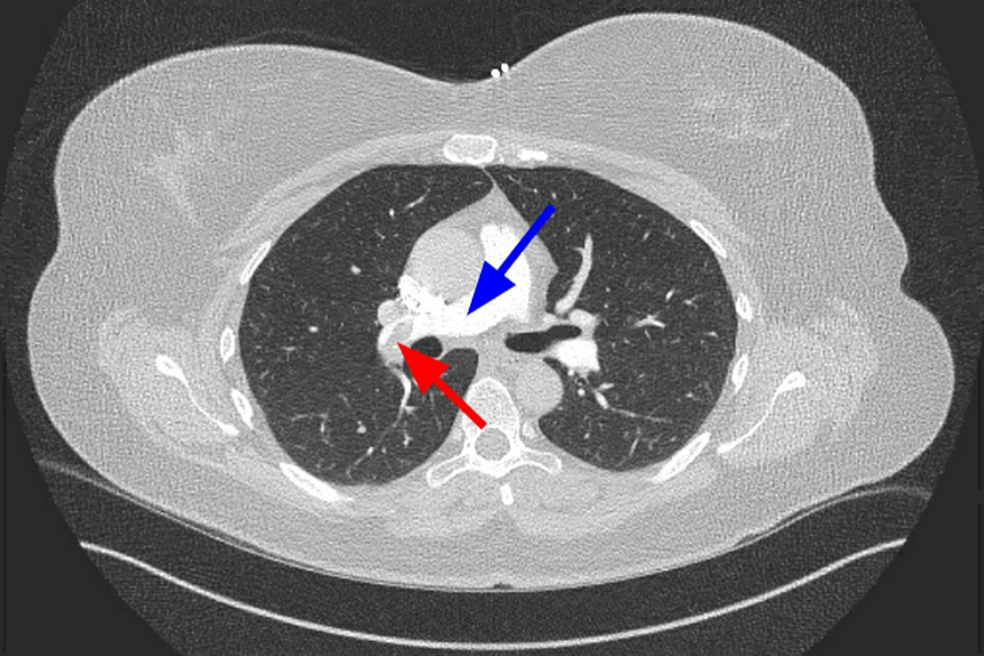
CTA PE protocol imaging showing right pulmonary artery (blue arrow) with thrombus in the distal right pulmonary artery (red arrow). CTA, computed tomography angiography; PE, pulmonary embolism
